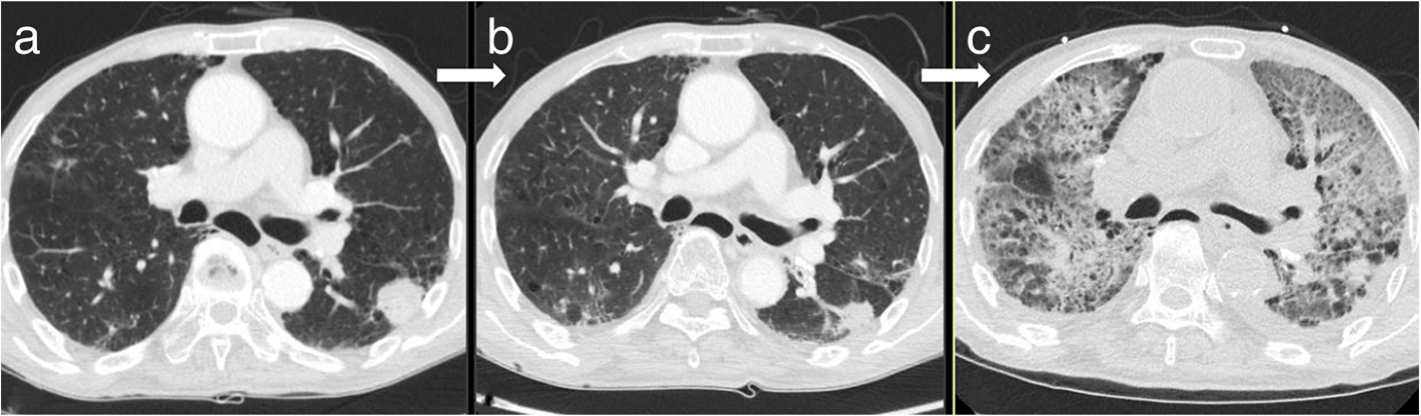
Chest CT axial imaging of the patient who showed grade 5 radiation pneumonitis after radiotherapy; (3a) Pretreatment chest CT image, (3b) At 1 month follow-up, and (3c) At 2 months follow-up
